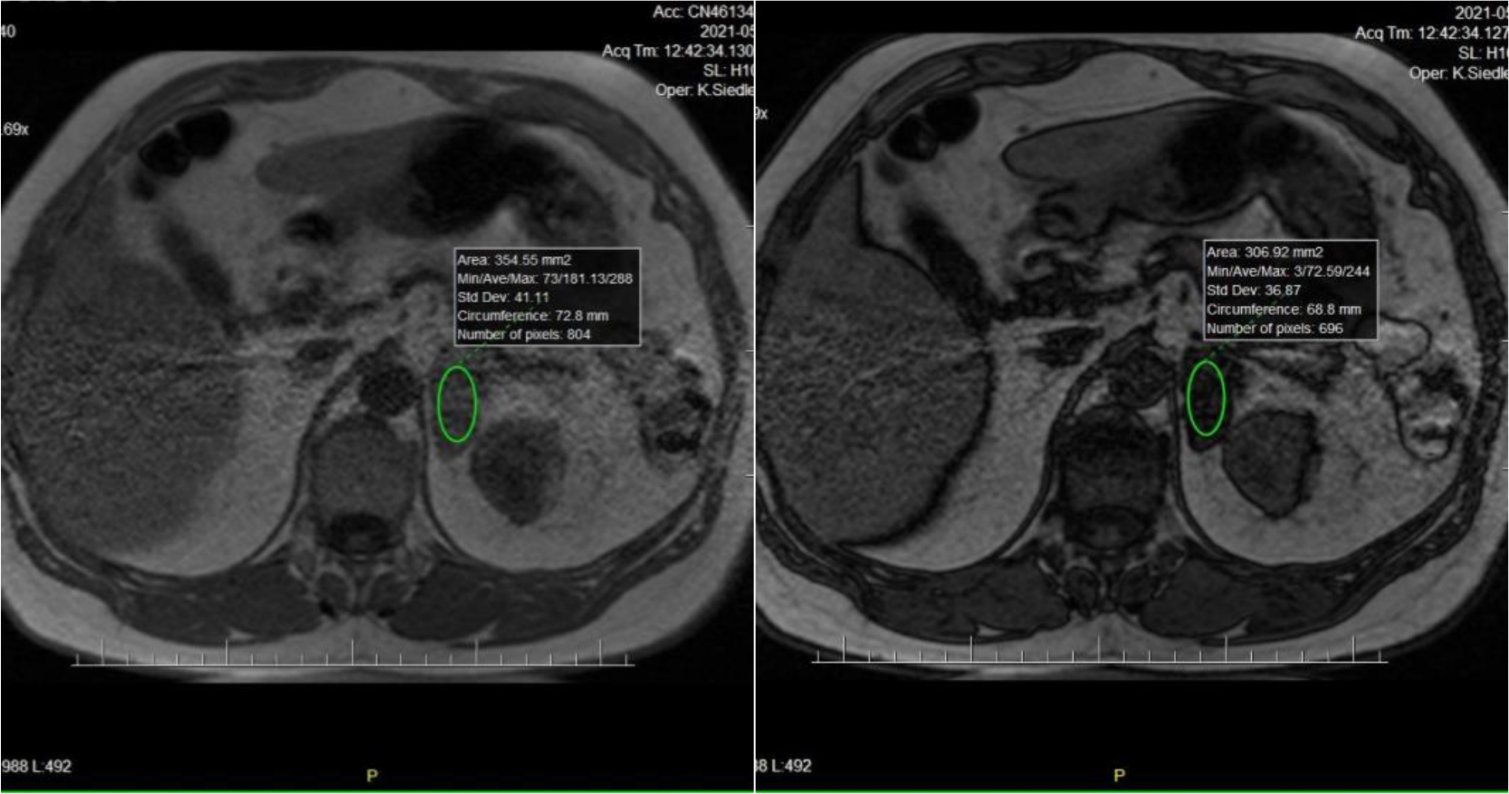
Adrenocortical carcinoma demonstrating signal loss on out-of-phase chemical shift MRI.
